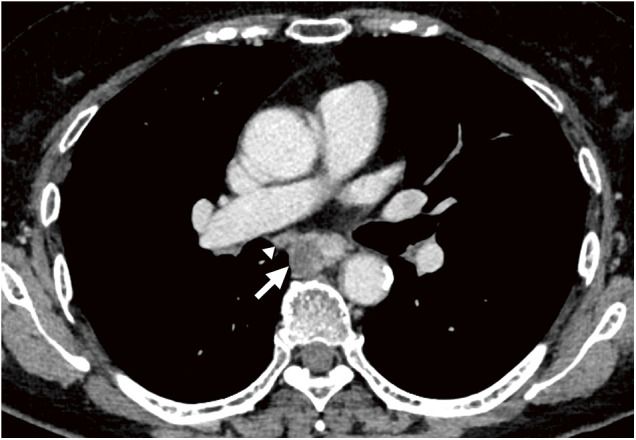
CT findings of primary lesion before neoadjuvant chemotherapy treatment. CT shows a thick wall around the middle thoracic esophagus (arrow) and enlargement of subcarinal LNs suspected of metastasis (arrowhead). CT: computed tomography; LN: lymph node
